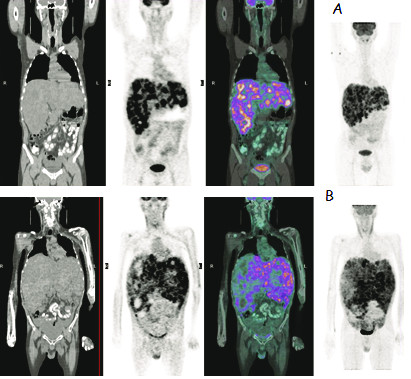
39 years-old male patient who received 1.6 GBq Y-90 microsphere therapy to the right and left lobe in separate sessions for primary hemangioendothelioma of the liver. 5A; coronal CT, 18F-FDG PET, fused and maximum intensity projection images of the whole body before the treatment. 5B; coronal CT, 18F-FDG PET, fused and maximum intensity projection images of the whole body after the treatment.
